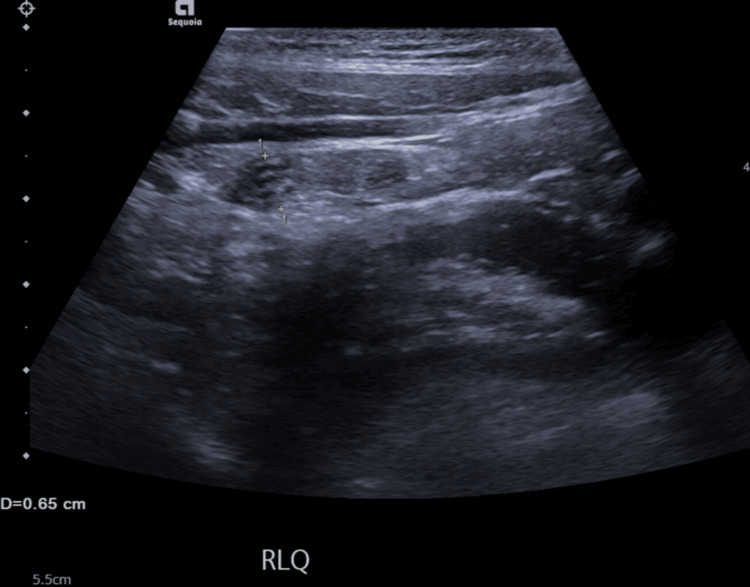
Ultrasound scan of a 12-year-old female from the study, where the right lower abdomen shows a blind-ending tubular structure measuring 6.5 mm, consistent with a dilated appendix. Typical ultrasonographic findings of appendicitis include a diameter ≥6 mm, wall thickening, absent peristalsis, and periappendiceal fat changes. Image Credit: The figure is original and derived from the data collected in the present study.
